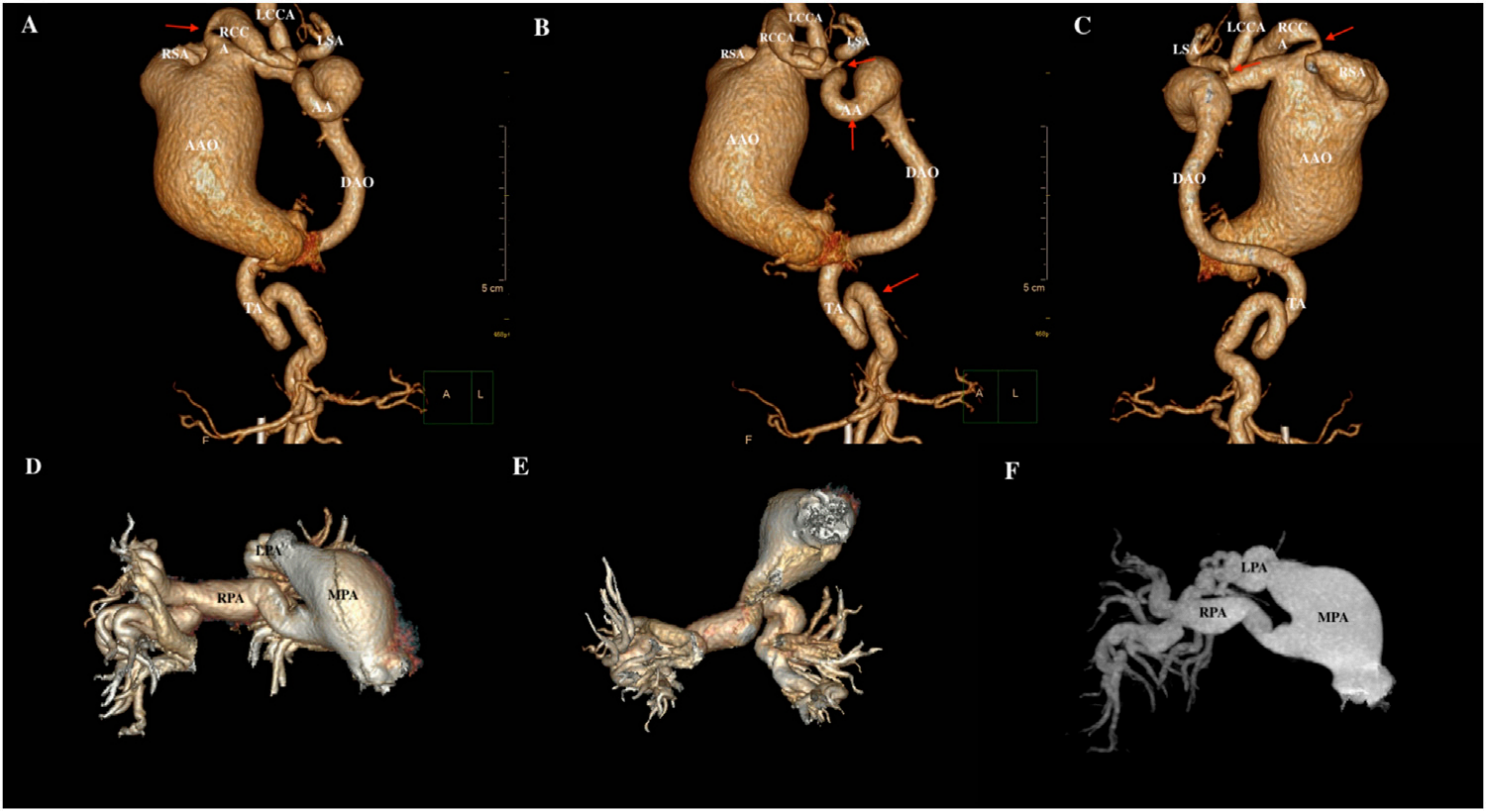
The CTA results of cardiac and great vessels. (A–C) The ascending aorta was severely dilated with branch tortuosity and stenosis (red arrow), and arcus transverse aorta tortuosity (red arrow) with aneurysmal dilatation. (D–F), Dilated, curved main pulmonary artery and its branches. RSA, right subclavian artery; RCCA, right common carotid artery; LCCA, left common carotid artery; LSA, left subclavian artery; AA, aortic arch; AAO, ascending aorta; DAO, descending aorta; TA, thoracic aorta; MPA, main pulmonary artery; RPA, right pulmonary artery; LPA, left pulmonary artery.
